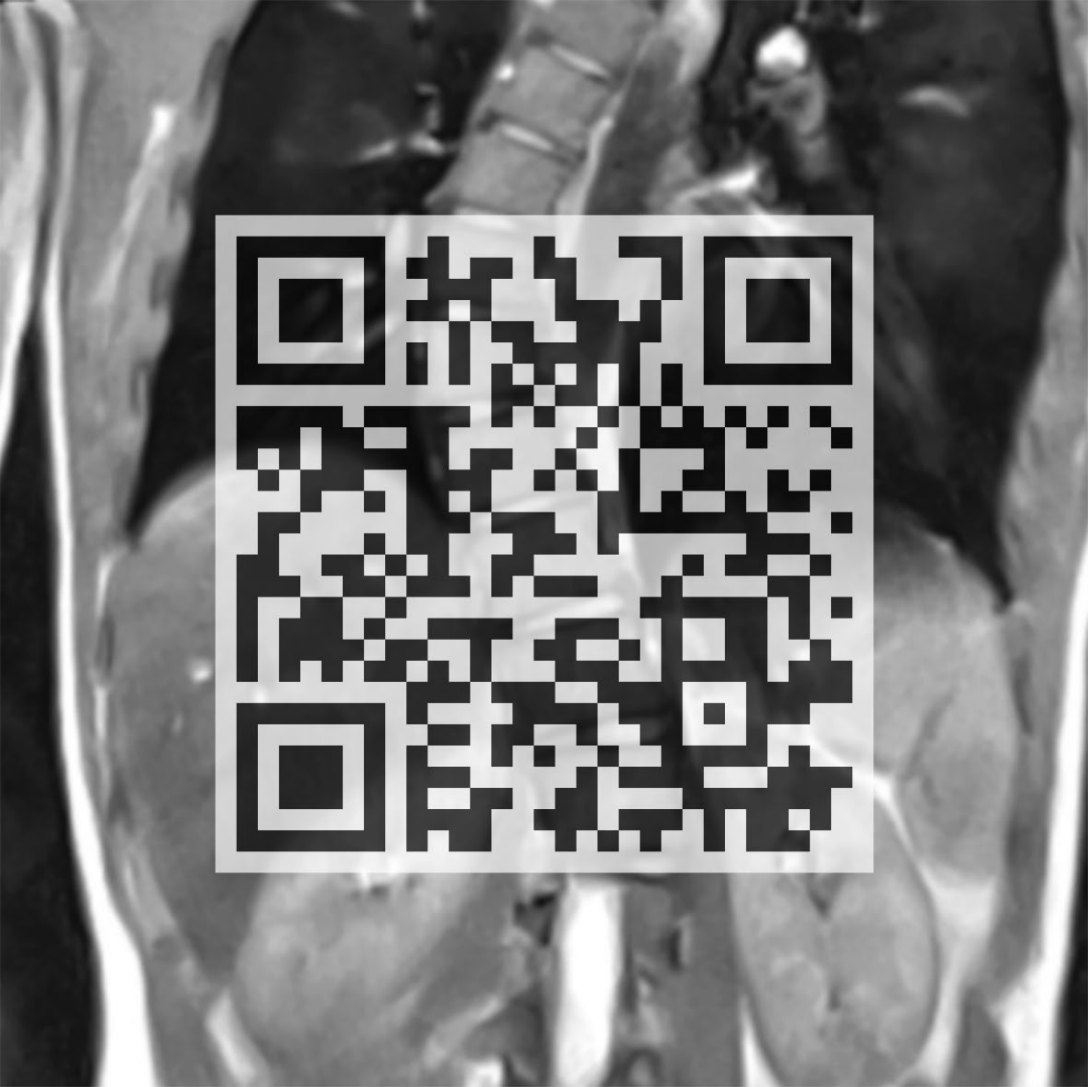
Video 12 T1/T2-weighted volume coverage video of a whole spine in a twelve-year-old boy with scoliosis. The total acquisition time for the three stacks was 40 seconds. Subsequently, a video of the whole spine is composed with a standard MRI stitching tool
